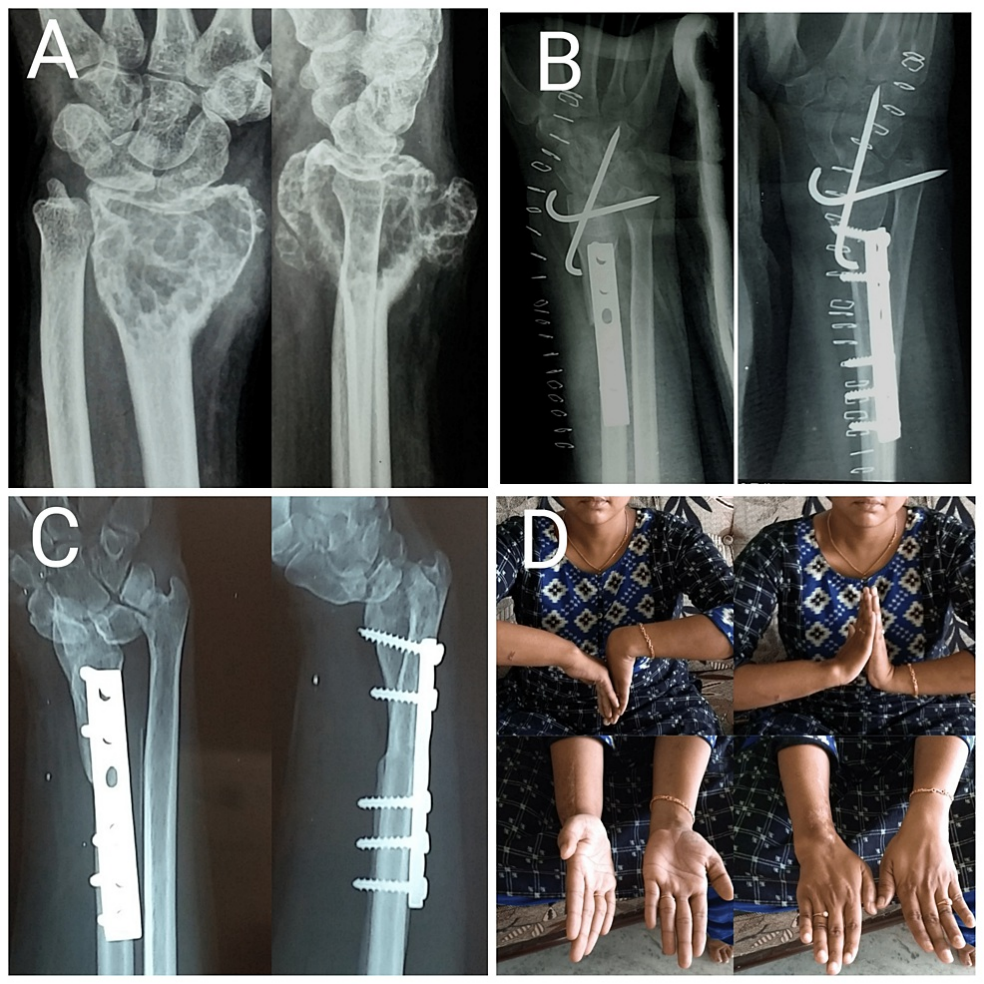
Case images of a 23-year-old female patient with Campanacci grade III giant cell tumor of right distal radius (A) Preoperative radiograph showing giant cell tumor of distal radius; (B) Immediate postoperative radiograph showing wide excision and ipsilateral proximal fibular autograft; (C) One-year post-operative radiograph showing union at fibuloradial junction with subluxation at wrist joint; (D) Reduced forearm rotations but good wrist and hand function after fibular autograft.
